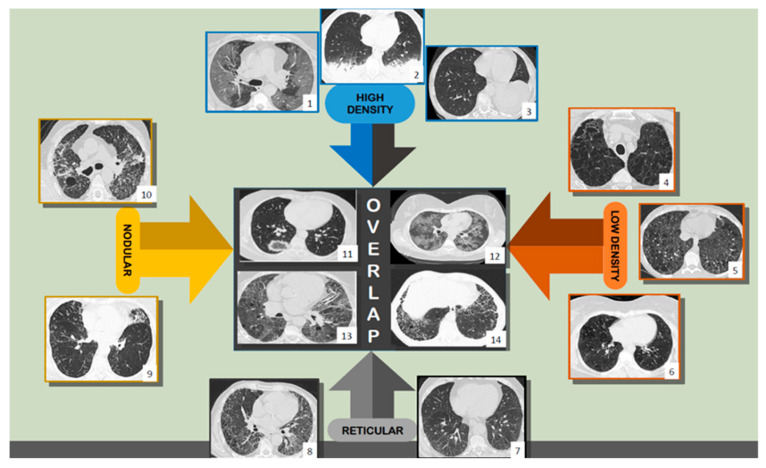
Axial thin-section CT scans, injury patterns: high density (1, 2, 3), low density (4, 5, 6), reticular (7, 8), nodular pattern (9, 10), and overlapping (11, 12, 13, 14). Scans belong to the ‘Dr. Victor Babes’ Infectious Diseases and Pneumoftiziology Clinical Hospital Timisoara database.
